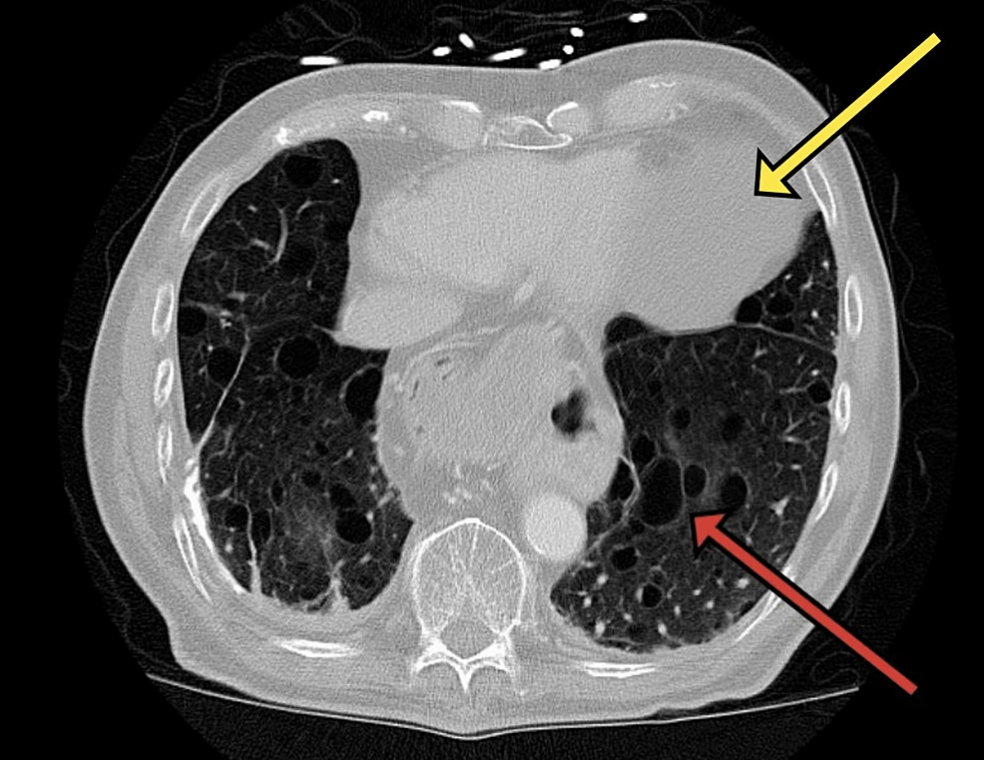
CT scan of the chest (transverse section of lung) showing one of the larger cysts (red arrow) in the background of cystic lung disease, and the pericardial cyst (yellow arrow).
